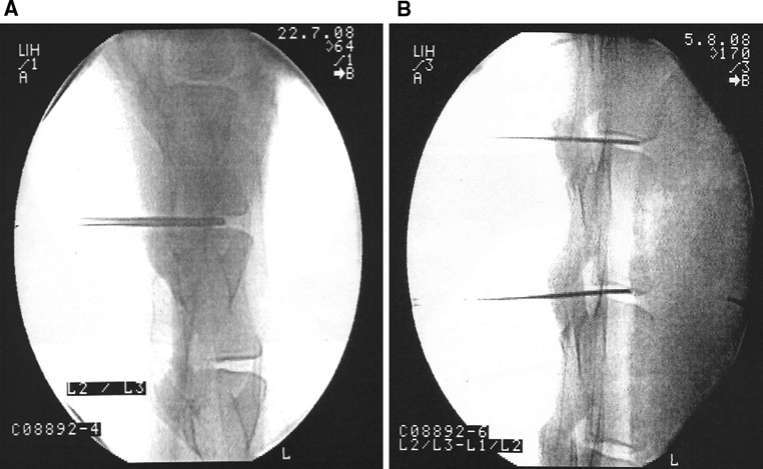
a C-arm X-ray image. 4-mm biopsy needle positioned into disc L2-3. The radiological image is a print out from the C-arm instrument, *perpendicular view*. b C-arm image: 18 gauge needles positioned into L1-2 and L2-3 prior to the injection
